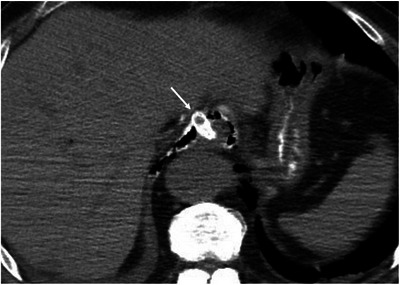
Mediastinitis after EUS‐HGS. During EUS‐HGS, the intrahepatic bile duct in segment 2 was punctured, resulting in the placement of a covered metal stent from the esophagus. Three days after the procedure, the patient developed a high fever, and computed tomography revealed air bubbles around the HGS stent in the mediastinum (→), suggesting mediastinitis. EUS‐HGS, endoscopic ultrasound‐guided hepaticogastrostomy.
